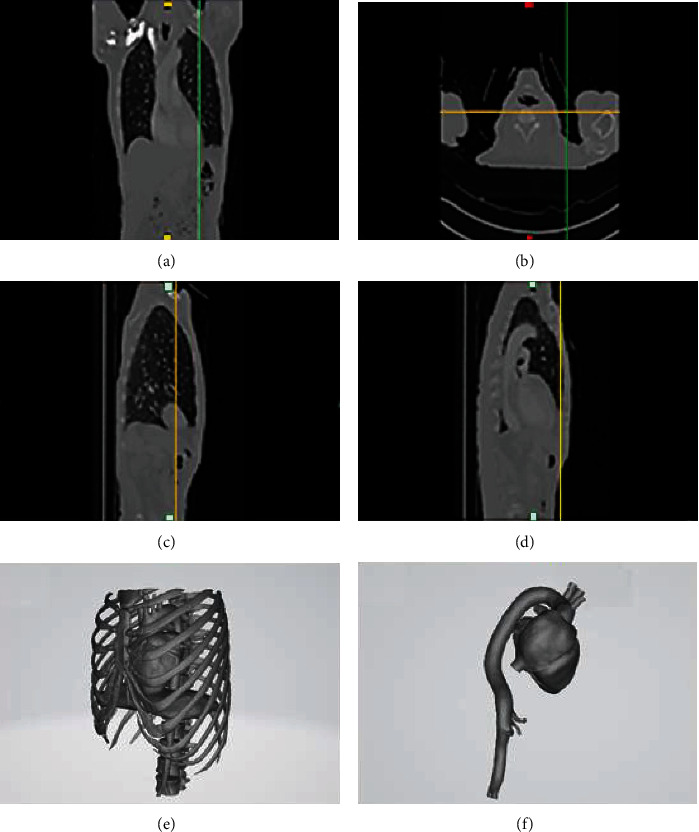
Modeling result of the whole chest in MIMICS (a) front view, (b) top view, (c, d) side views of chest MRI pictures, (e) general view of the whole model, and (f) general view of the aorta-heart model.
